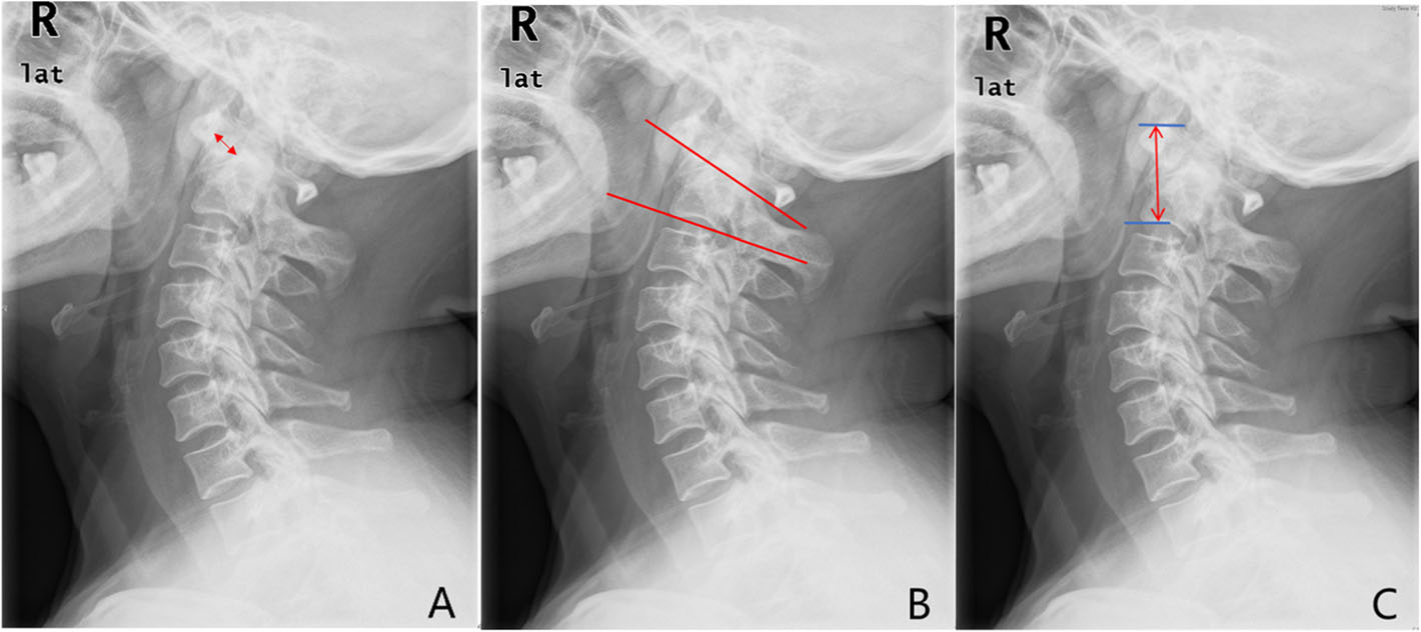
Radiographic parameters. a Atlas-dens interval (ADI): the distance between the posterior margins of the anterior arches of the C1 vertebra and the anterior margin of Odontoid process. b Atlantoaxial angle (A-A angle, the C1-C2 angle): the angle between the line connecting the lower margins of the anterior and posterior arches of the C1 vertebra and the lower margin of the C2 vertebra. c Atlantoaxial height (A-A height): the distance between the upper margin of the anterior arch of the C1 vertebra and the lower margin of the C2 vertebral body
